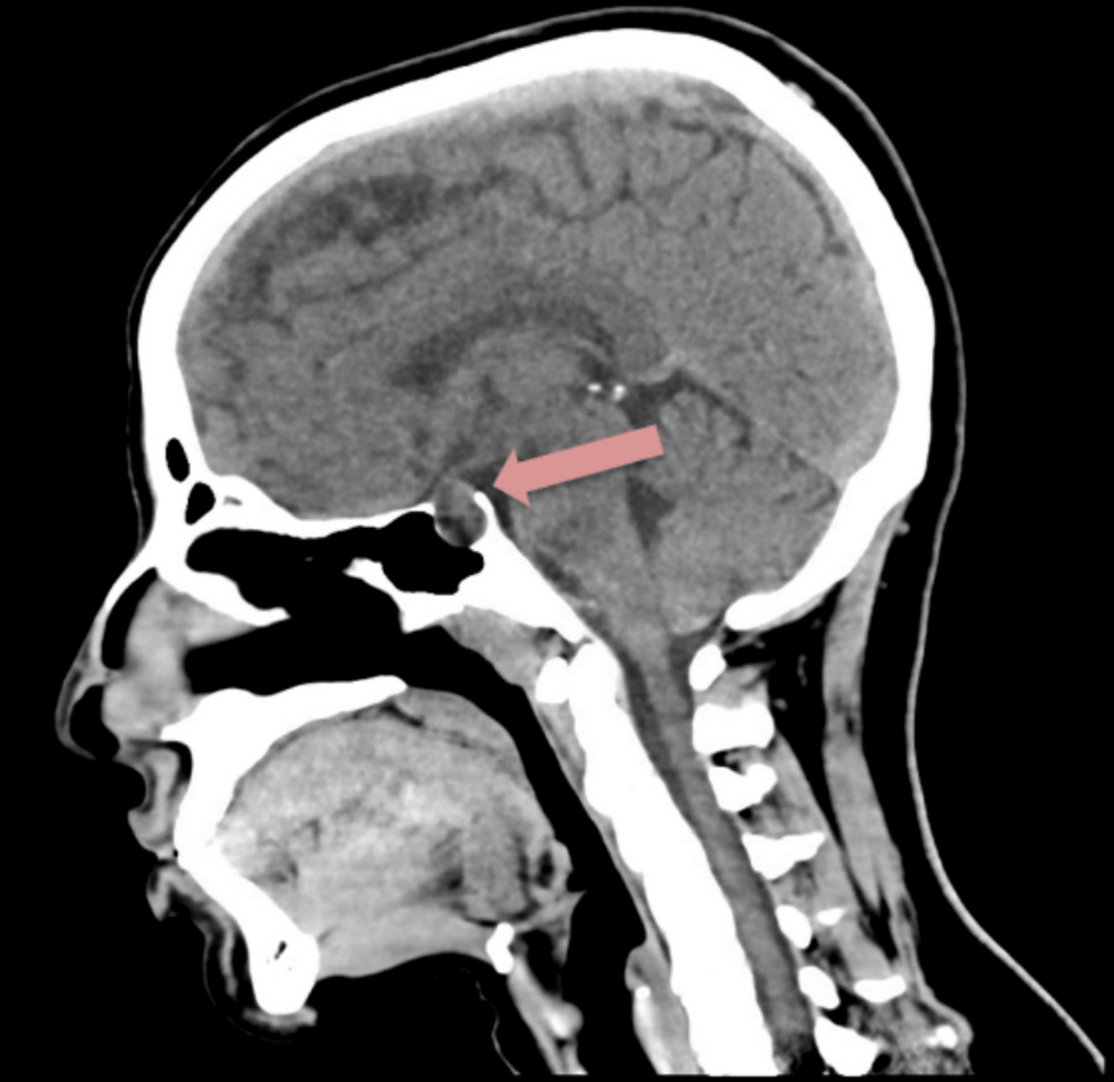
Preoperative navigation computed tomography showing a hemorrhagic sellar lesion with suprasellar extension (arrow). The scan demonstrates a heterogeneous sellar mass with suprasellar extension and mild displacement of the optic chiasm, consistent with pituitary apoplexy.
